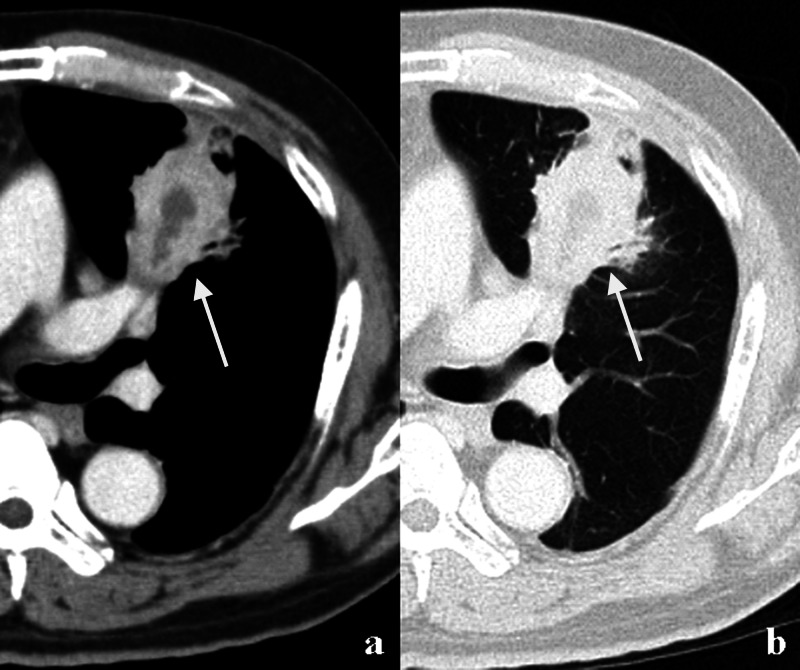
Enhanced chest CT The enhanced chest CT showed a 4.0 cm mass-like lesion in the lingular segment of the left lung. (a) Soft tissue density image and (b) lung density image. There was slight pleural thickening, and it was suspected that inflammation had spread to the pleura.
